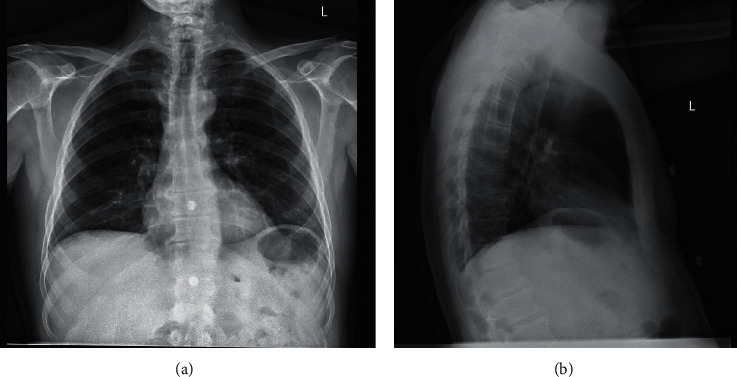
Frontal (a) and lateral (b) chest X-ray images demonstrating no abnormalities or signs of pericardial effusion.
